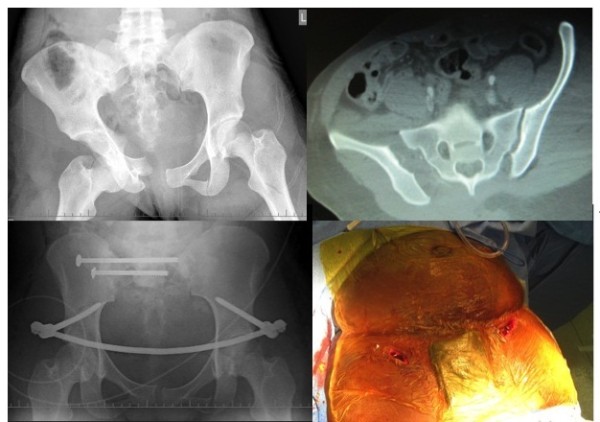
Case Example a) Xray APC 3 Pelvic injury b) CT scan showing opening of Right SI joint c) Fixation with posterior SI screws and INFIX anteriorly d) Incisions for insertion of INFIX before closure.
